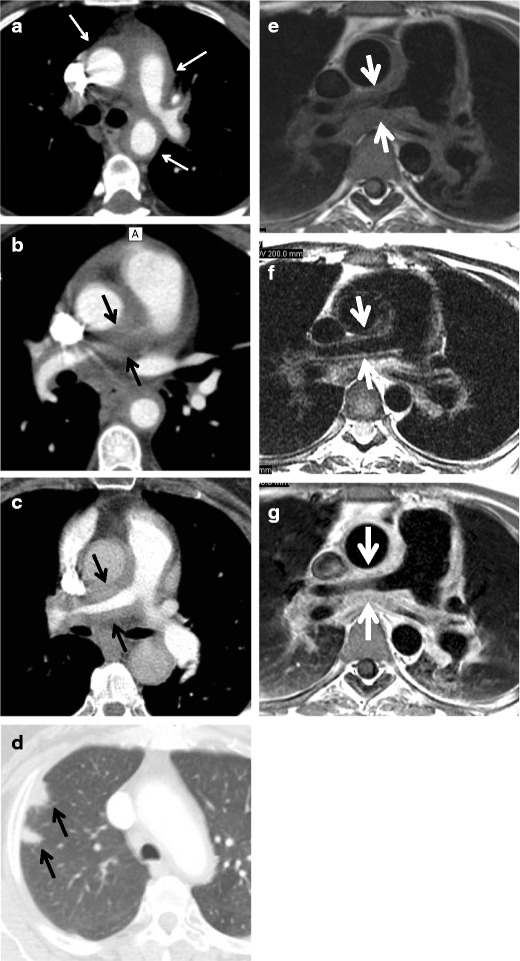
a–g A 63-year-old man with a prolonged history of Takayasu arteritis. MDCTA axial images of the chest at the level of the main and left pulmonary artery (a), right pulmonary artery (b, c) and *right upper* lobe demonstrate substantial thickening of the pulmonary arterial wall, especially pronounced at the level of the right pulmonary artery with almost complete occlusion of the *right upper* lobe branch and pulmonary infarcts (d) (*arrows*). T1-weighted (e), T2-weighted (f) and GD-enhanced (g) MRI images emphasise the same findings as well as substantial wall oedema seen as bright signal on T2-weighted images (f) (*arrows*)Both contrast-enhanced MRI and CTA play an important role in the early diagnosis of TA, i.e., by demonstrating crescentic or ring-like aortic thickening of more than 3 mm, as well as for assessing disease activity [32].Limitations of MR angiography include the possibility that vascular branch points may be improperly interpreted as occlusions (breath-hold techniques have lessened this problem) and that maximum-intensity projection images may falsely accentuate the degree of vascular stenosis [5]. It is therefore advisable to assess the degree of vascular stenoses from the MR angiography source images [18]. Additional drawbacks of MRI are the relatively decreased sensitivity in the assessment of small vessels and poor visualisation of vascular calcifications, as well as the limited anatomical range that can be scanned as opposed to CT. Finally, MRI is commonly less available in the geographic regions where TA is most prevalent [18].Nuclear imagingPET-CT may help to identify vasculitis in patients referred for whole-body imaging for constitutional symptoms and fever of unknown origin, as well as to monitor treatment response [11]. The circumferential region of increased metabolic activity in the vessel wall is characteristic of the active phase of TA (Fig. 7). FDG-PET has a reported sensitivity of up to 92 % and a specificity of 89–100 % for the detection of large vessel vasculitis among untreated patients with elevated serum markers [33]. Recently, the use of 18-fluorodeoxyglucose (18F-FDG) PET, either alone or in combination with contrast-enhanced CTA or MRA, has emerged as a potential tool for the initial diagnosis and assessment of disease activity of aortitis caused by Takayasu arteritis with a variable sensitivity of 60–90 % and specificity of 88–100 % [24, 13, 12, 34]. PET-CT may also be useful for monitoring treatment response, reflected in decreases in vessel wall metabolic activity [35]. However, the main limitations of these studies are the small sample size, heterogeneous patient population and inconsistent reference standards [36].
